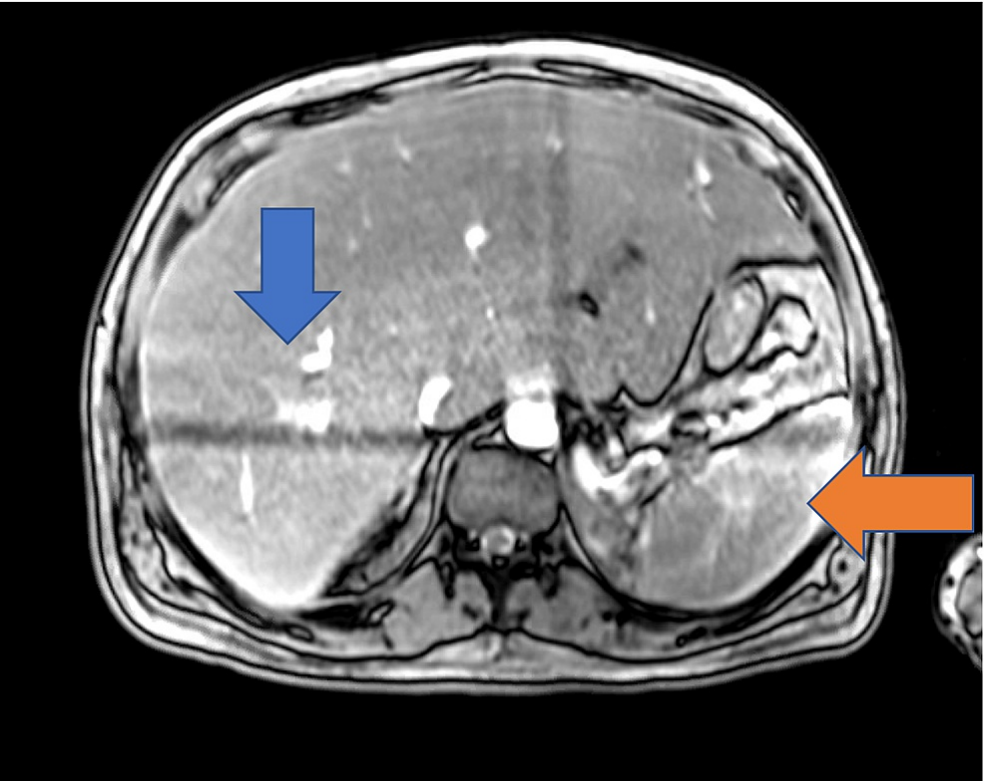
MRI of the liver with contrast Markedly enlarged liver with abnormal T2 signal (blue arrow) and heterogeneous enhancement with splenomegaly (orange arrow).
